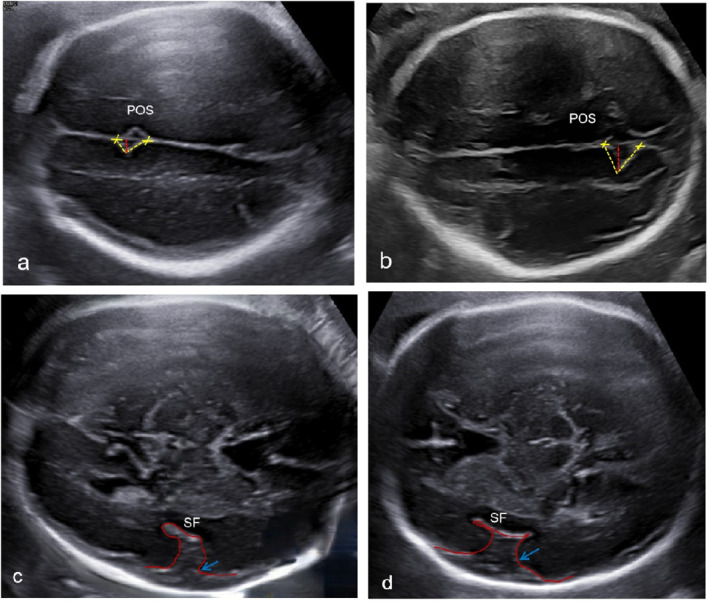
Ultrasound images of the Sylvian fissure (SF) and parieto‐occipital sulcus (POS) at 29 weeks in family 1 fetus compared to a fetus with a normal neurological system. (a) The POS of family 1 fetus at 29 weeks. (b) The POS of a fetus with a normal neurological system at 29 weeks. The POS depth of the fetus in family 1 is significantly lower than that of normal fetuses at 29 weeks (c) The SF of family 1 fetus at 29 weeks. (d) The SF of a fetus with a normal neurological system at 29 weeks.
